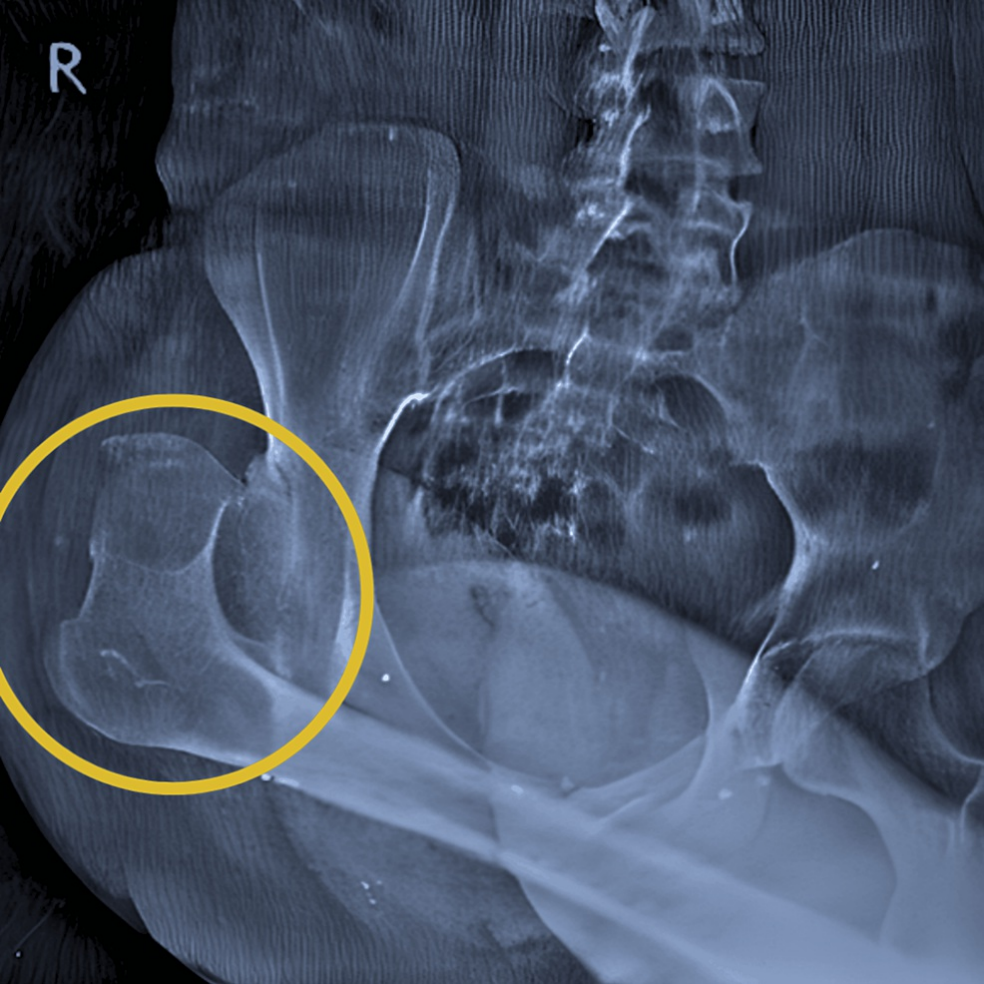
Anteroposterior view of radiograph shows suggestive of right femoral head dislocation The yellow circle shows the dislocation of the right femoral head.
